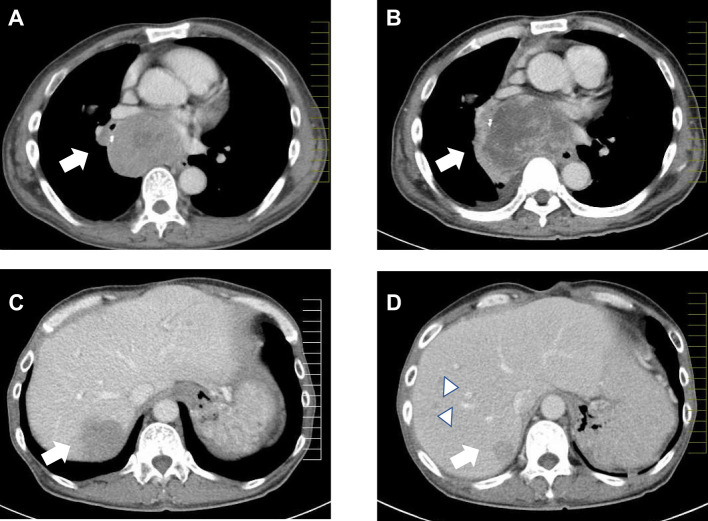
Computed tomography images of tumors in the mediastinum (A, B) and liver (C, D) of Patient II-1. The arrows indicate the main tumors. Comparing tumor size before the initiation of octreotide treatment and transcatheter arterial chemoembolization (A) and 10 months after treatment showed an enlargement of the posterior mediastinal tumor (B). The liver tumor in segment S7 shrunk after treatment (D) when compared to its size 10 months before then (C). Multiple new liver tumors were confirmed (D, arrowheads).
